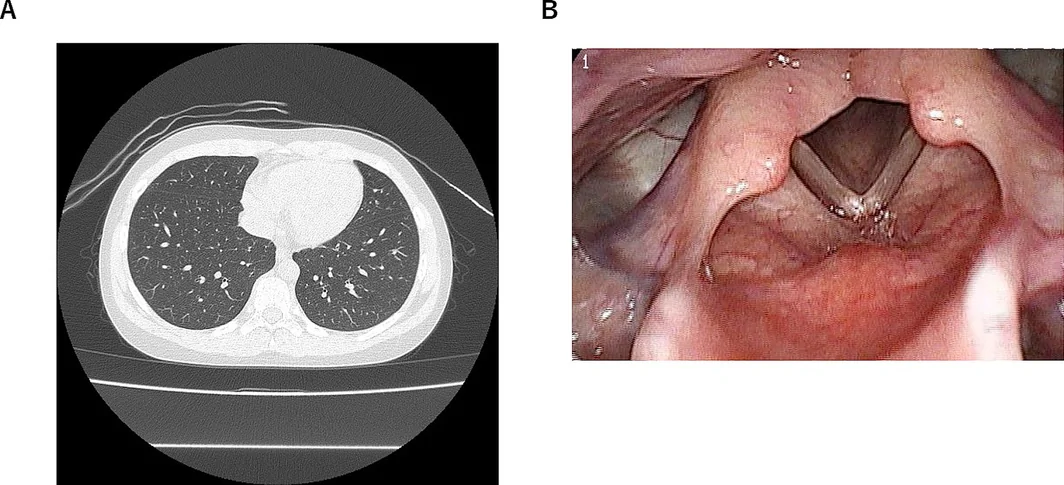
Chest computed tomography (A, March 2024) and Laryngoscopy (B, October 2023). Laryngoscopy was performed during asymptomatic and demonstrated no obstructive lesion in the upper airway.
